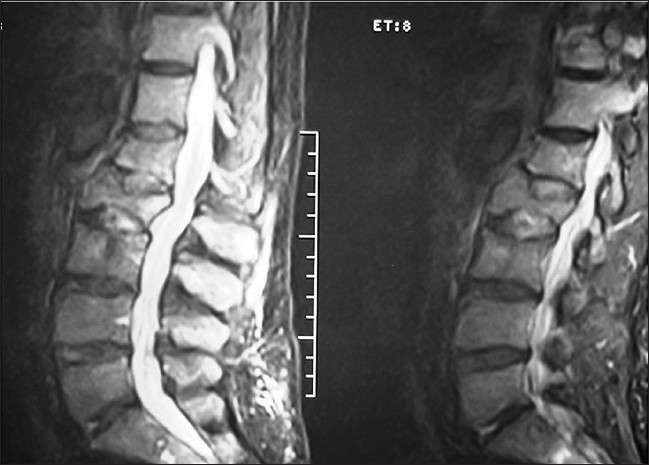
T2W1 of MRI of Lumbasacral spine shows compression fracture
